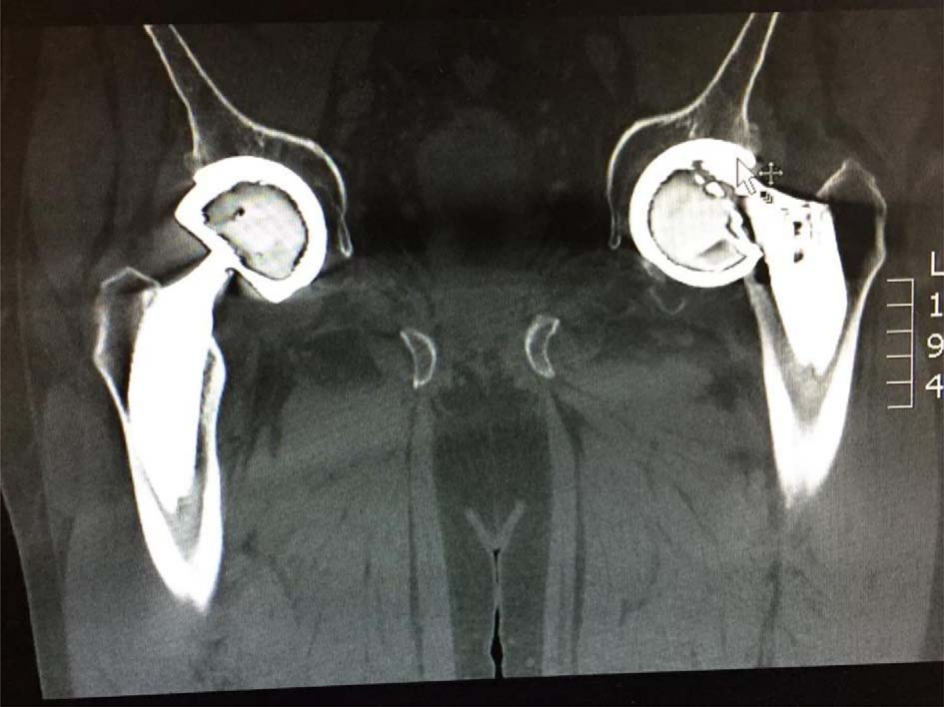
CT scan (Coronal View) showing dislocated femoral head for the trunnion of the femoral neck.
